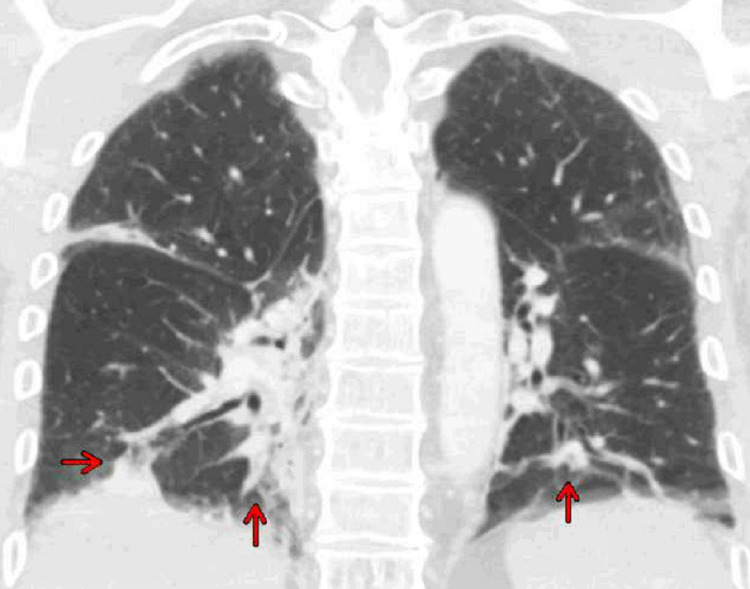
Computed tomography of the chest with contrast coronal view revealed bibasilar right greater than left consolidations and ground-glass opacities (red arrows) with subsegmental atelectasis suggestive of pneumonia.
